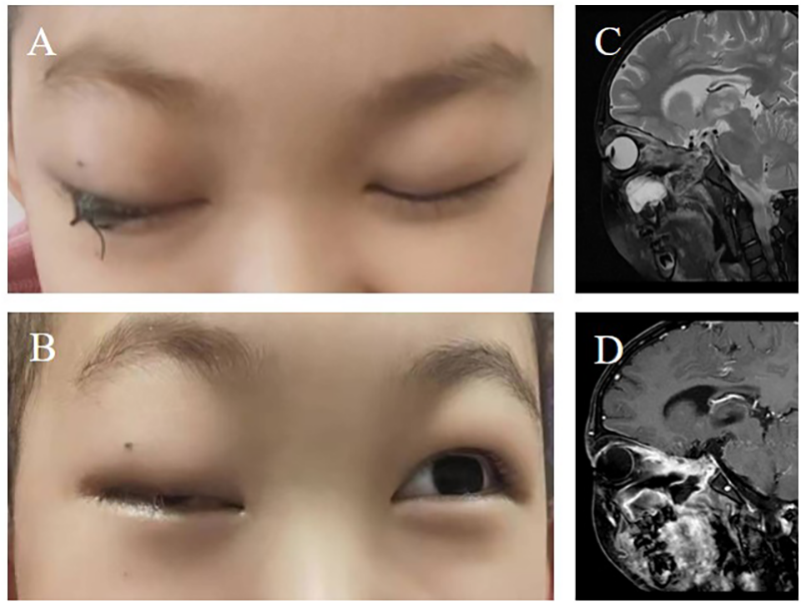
(A) Photographic representation of the tarsorrhaphy surgery performed to address the patient's visual complications. (B) At the 24-week postoperative follow-up, both eyes exhibited a lack of light perception, indicating persistent visual impairment. (C) Coronal and (D) axial views of contrast-enhanced magnetic resonance imaging of the head, conducted 24 weeks after surgery, provided a radiologic assessment of the patient's condition, showing the extent of recovery and ongoing changes in the affected areas.
